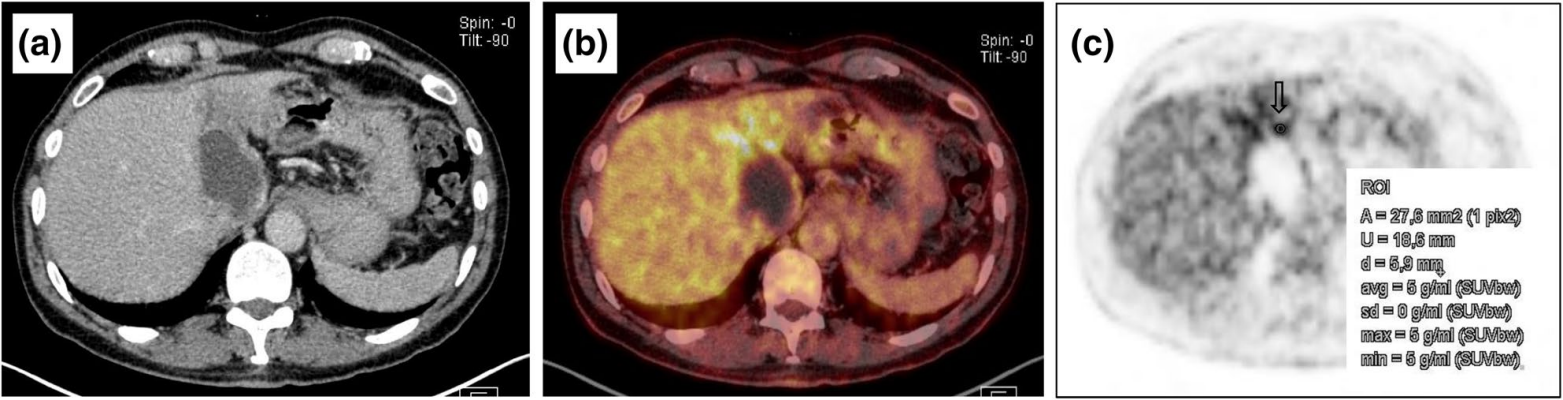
18-FDG PET/CT examination and measurement of SUV in an active hepatic alveolar echinococcosis lesion (EMUC-CT type I). (a) Contrast enhanced CT scan in the venous phase, (b) combined PET/CT, (c) quantitative measurement of the maximum SUV in a PET scan, for a defined ROI (arrow).
